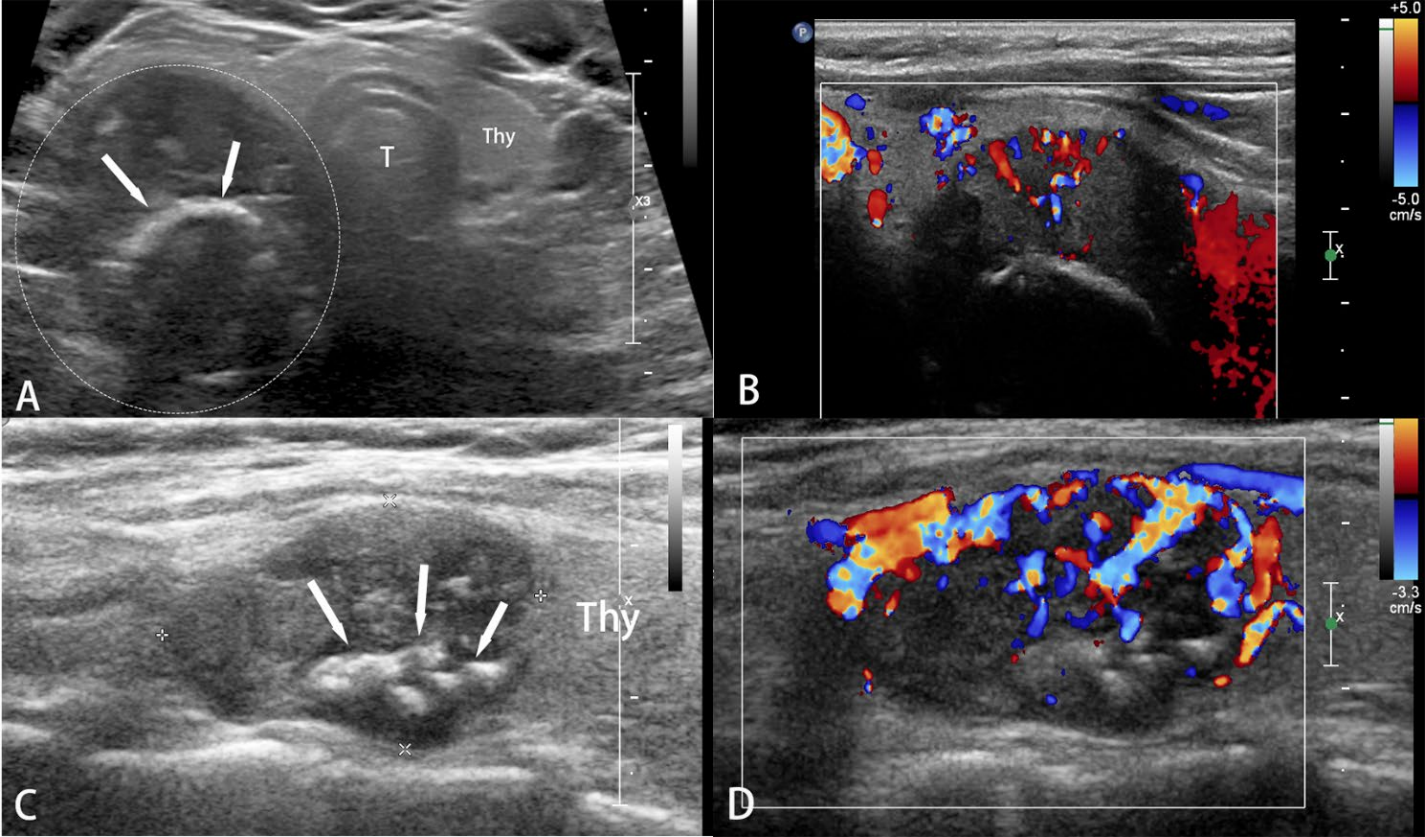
Calcification in the parathyroid carcinoma on ultrasonography. A Parathyroid carcinoma of a 45-year-old female with coarse calcification observed in the lesion (arrows); B Color Doppler ultrasound shows abundant blood flow in the lesion; C Parathyroid carcinoma of a 62-year-old female with multiple calcifications detected in the lesion (arrows); D Color Doppler ultrasound shows circumferential and internal blood flow in the lesion. Thy: thyroid; T: trachea
